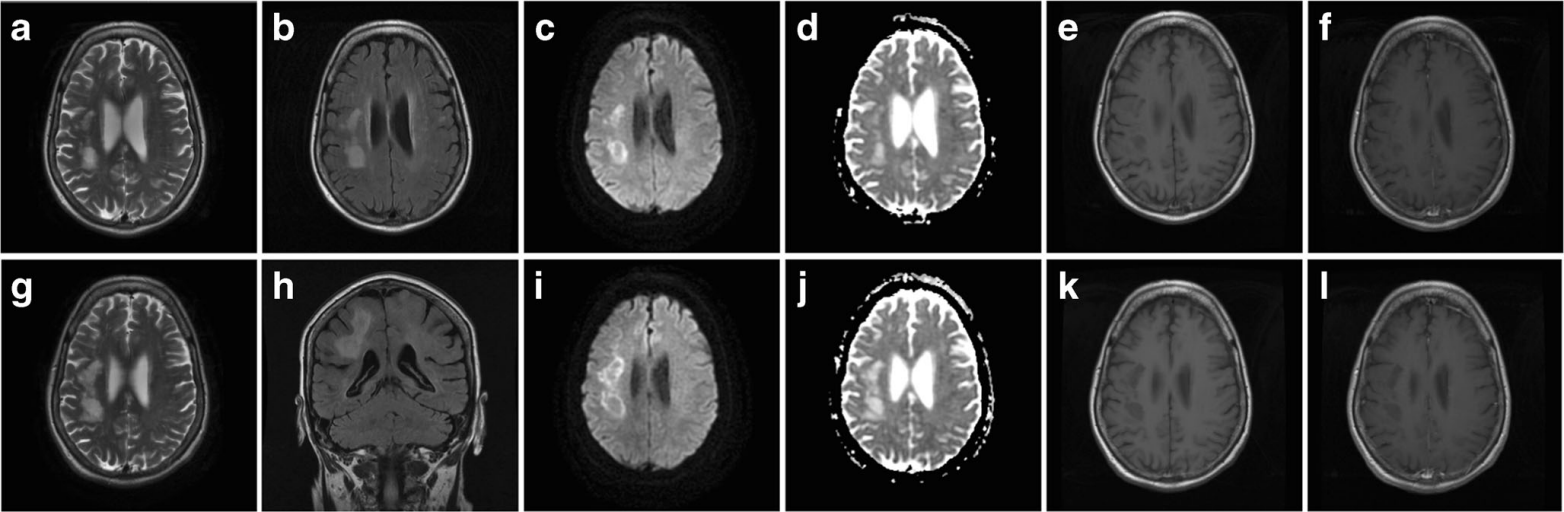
MR imaging of brain demonstrating multifocal areas of high signal on T2 (a, g) and flair (b, h) with restricted patchy and peripheral diffusion on the axial DWI sequence (c, i) matched on the ADC (d, j). T1 images showed low signal (e, k) and post-contrast (f, l) there was no enhancement. The imaging changes progressed over a one-month interval (a–f followed by g–l)
